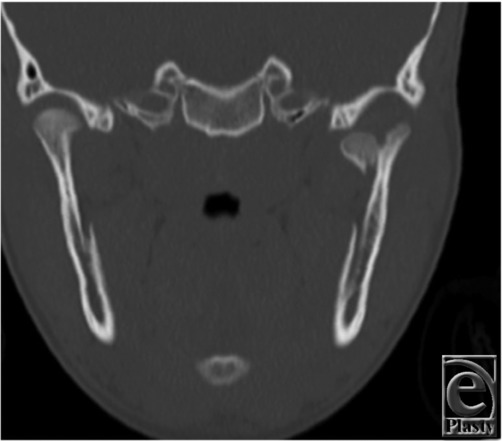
Maxillofacial computed tomographic scan, coronal cut, shows a displaced intracapsular left condylar fracture.
